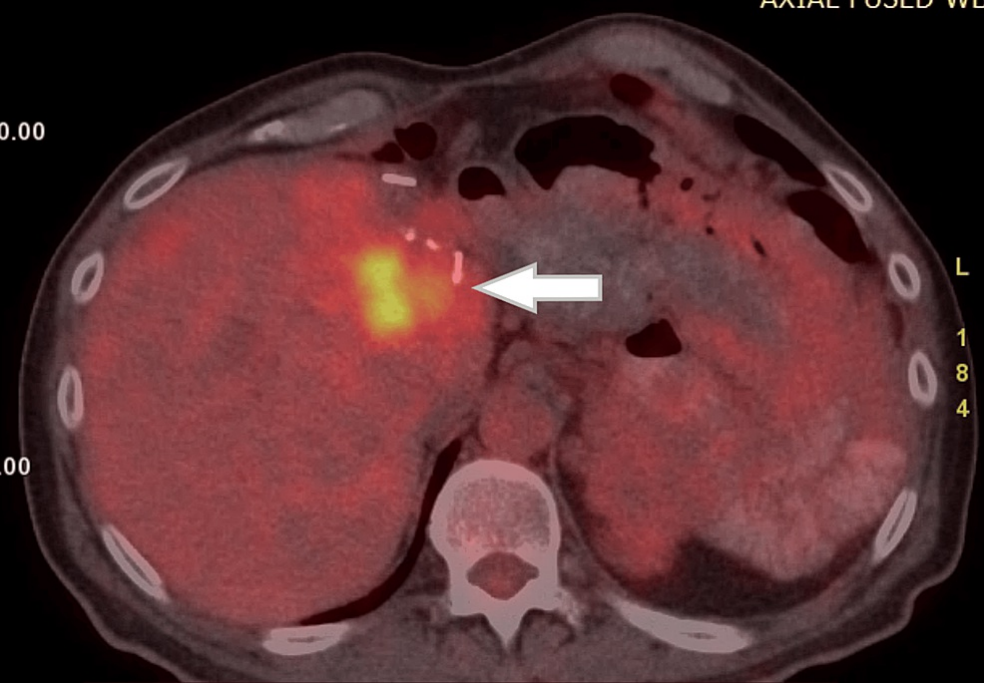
Axial PET-CT image of the abdomen The white arrow demonstrates liver disease recurrence. PET-CT: positron emission tomography-computed tomography
